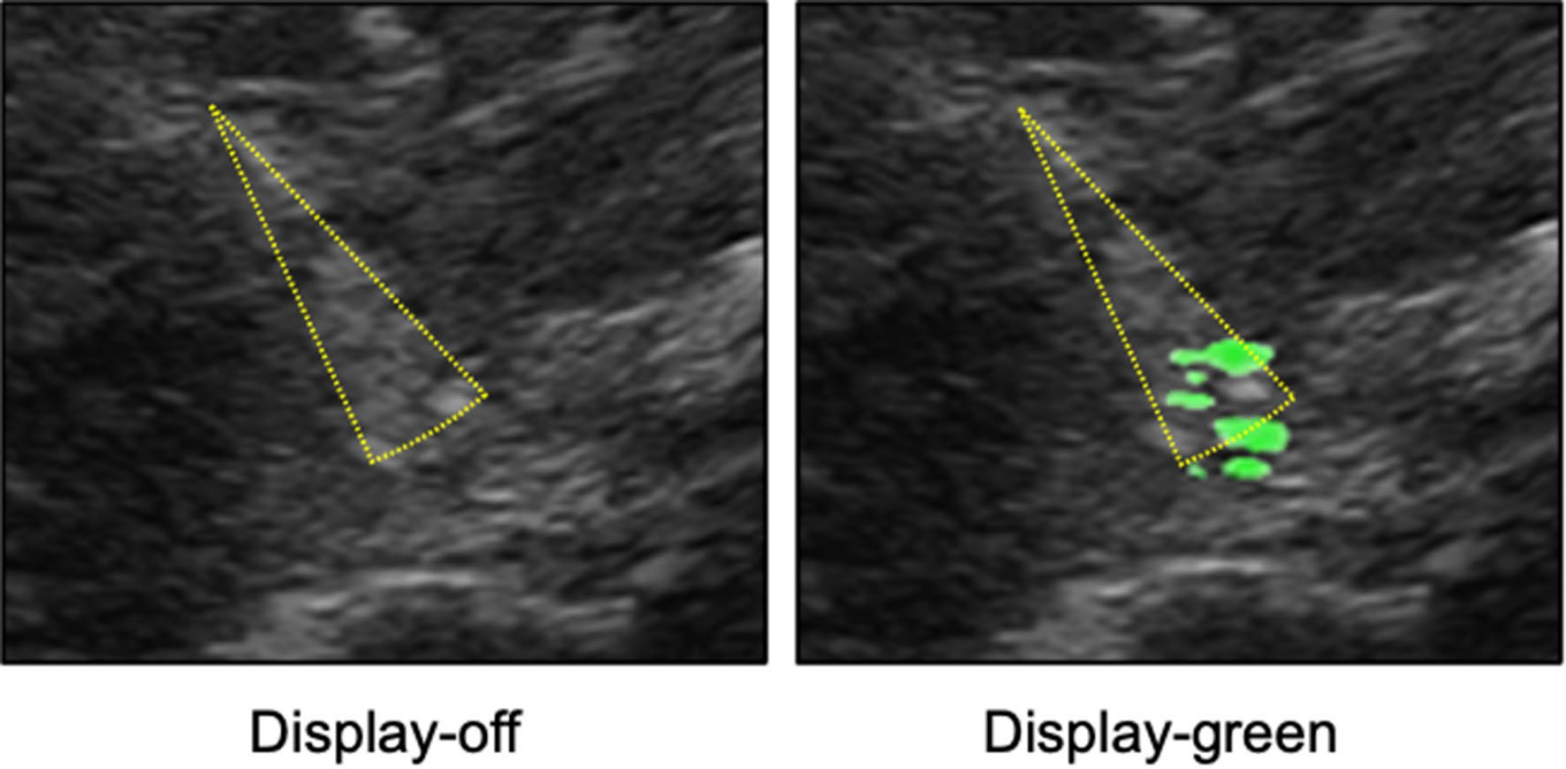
Artifacts cause multiple needle tips. Sciatic nerve block on the left side of the cadaver (See Supplemental Digital Content nos. 11 and 18). Combination of reverberation artifact and bayonet artifact (dotted yellow triangle) may cause an illusion of multiple needle tips (display-green; Supplemental Digital Content no. 18). The needle tip without displaying photoacoustic ultrasound cannot be identified (display-off; Supplemental Digital Content no. 11). Display-off: no photoacoustic ultrasound, display-green: photoacoustic ultrasound using green color.
